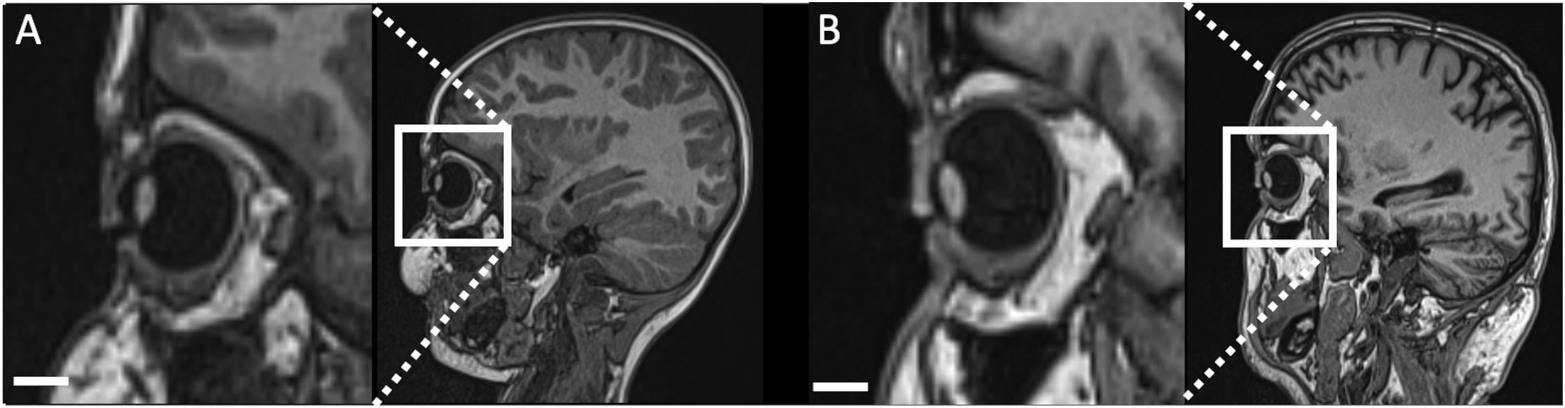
Representative examples of unenhanced T1-weighted 3D MPRAGE brain images in the sagittal plane of a 4-year-old female patient (A) and a 65-year-old male patient without hypertension (B) with the right eye magnified in the left panel (bar ≙ 7 mm).
